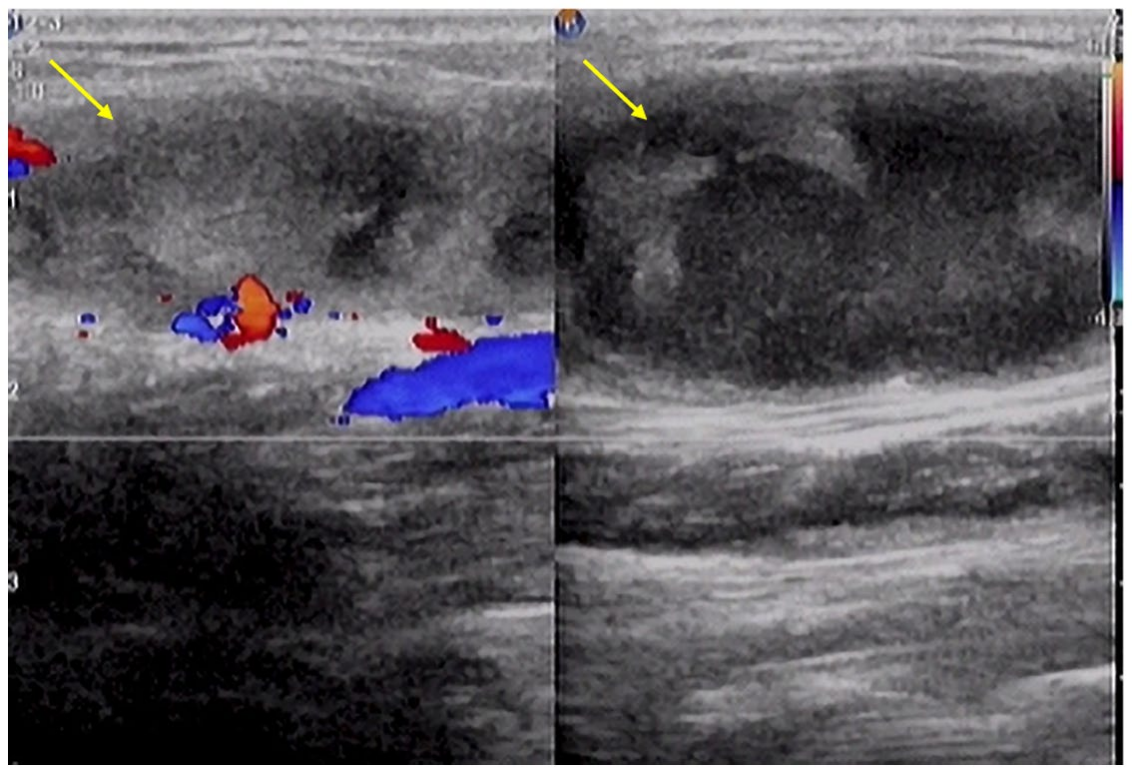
Ultrasonography reveals an irregular mass (arrows) with unclear edge.
